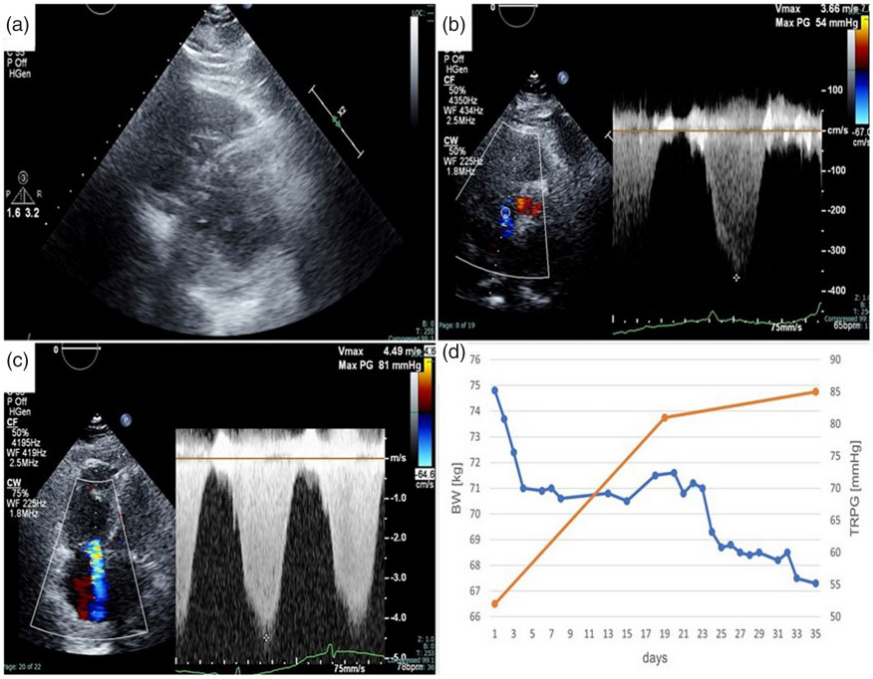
Trans-thoracic echocardiography (TTE) revealed right ventricular dilatation with flattening of the interventricular septum (*A*) and increased tricuspid regurgitation pressure gradient (54 mmHg) (*B*). Follow-up TTE on Day 19 revealed worsened tricuspid regurgitation pressure gradient (81 mmHg) (*C*) despite the patient losing body weight due to diuretic therapy (*D*).
